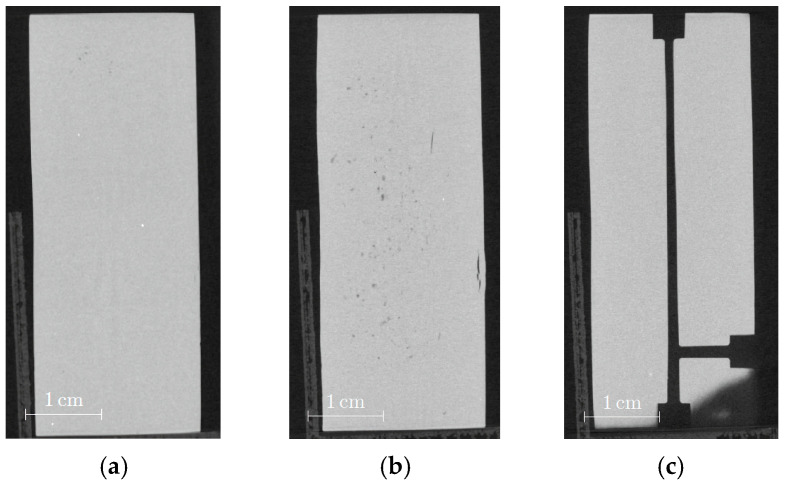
CT images of sintered plates: (a) unstructured plate close to the surface; (b) unstructured plate with a cross section in the middle; (c) structured plate close to the surface.
